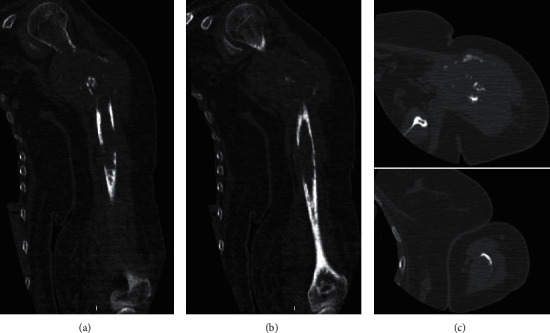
Preoperative CT scans demonstrating a large extraskeletal mass in the proximal left humerus causing a pathologic fracture, while a second lytic lesion is visible distally. On the right side: in the upper and lower corner, axial CT scans of the proximal and distal lesions are visible, respectively. (CT: computed tomography).
